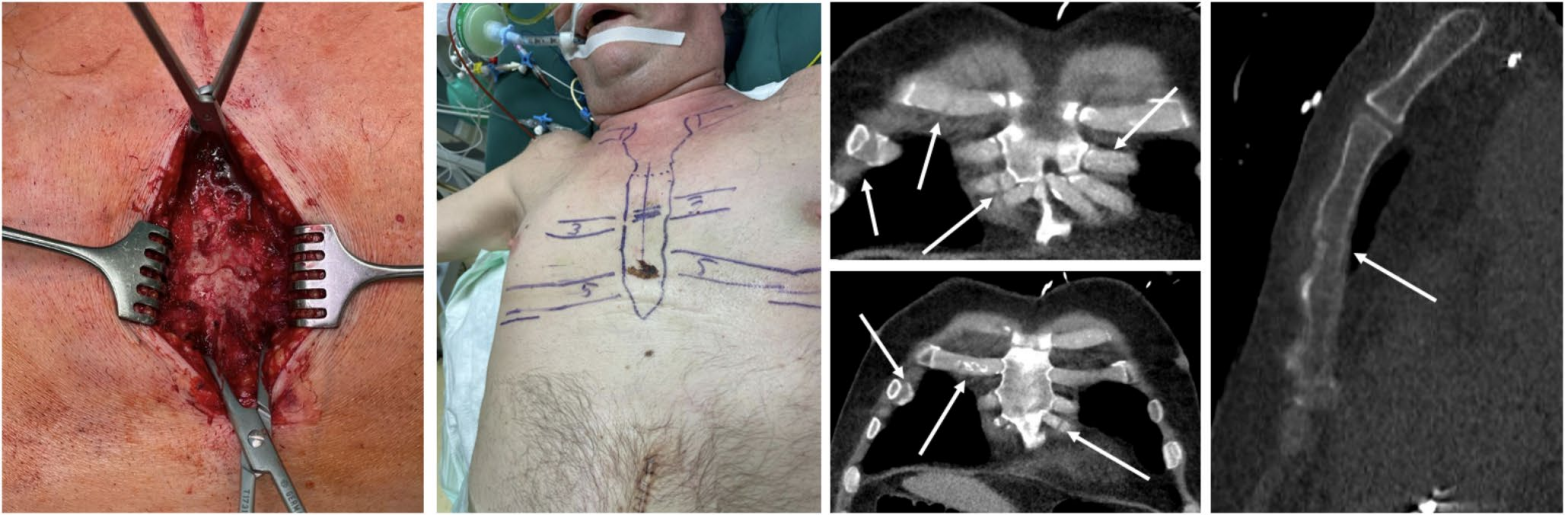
The standard fracture pattern is an undisplaced sternal fracture (left picture) and the osteochondral parasternal dissociation both sides, which can be difficult to detect in CT-diagnostics. The fracture pattern is then “mapped” on to the patient, to plan the typical three incisions, as shown in the picture in the middle
